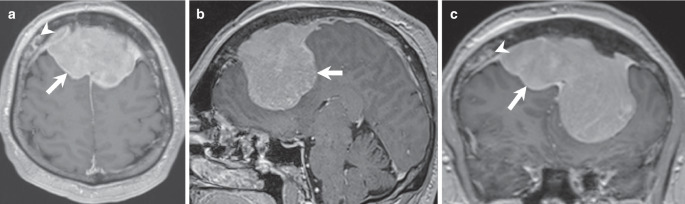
Axial (a), coronal (b), and sagittal (c) T1-weighted images, acquired post-gadolinium (Gd) administration, showed uniform contrast enhancement of the lesion (a–c, arrow). The osteolytic areas seen on the CT scan also showed homogeneous enhancement of contrast (a, c, arrowhead)
